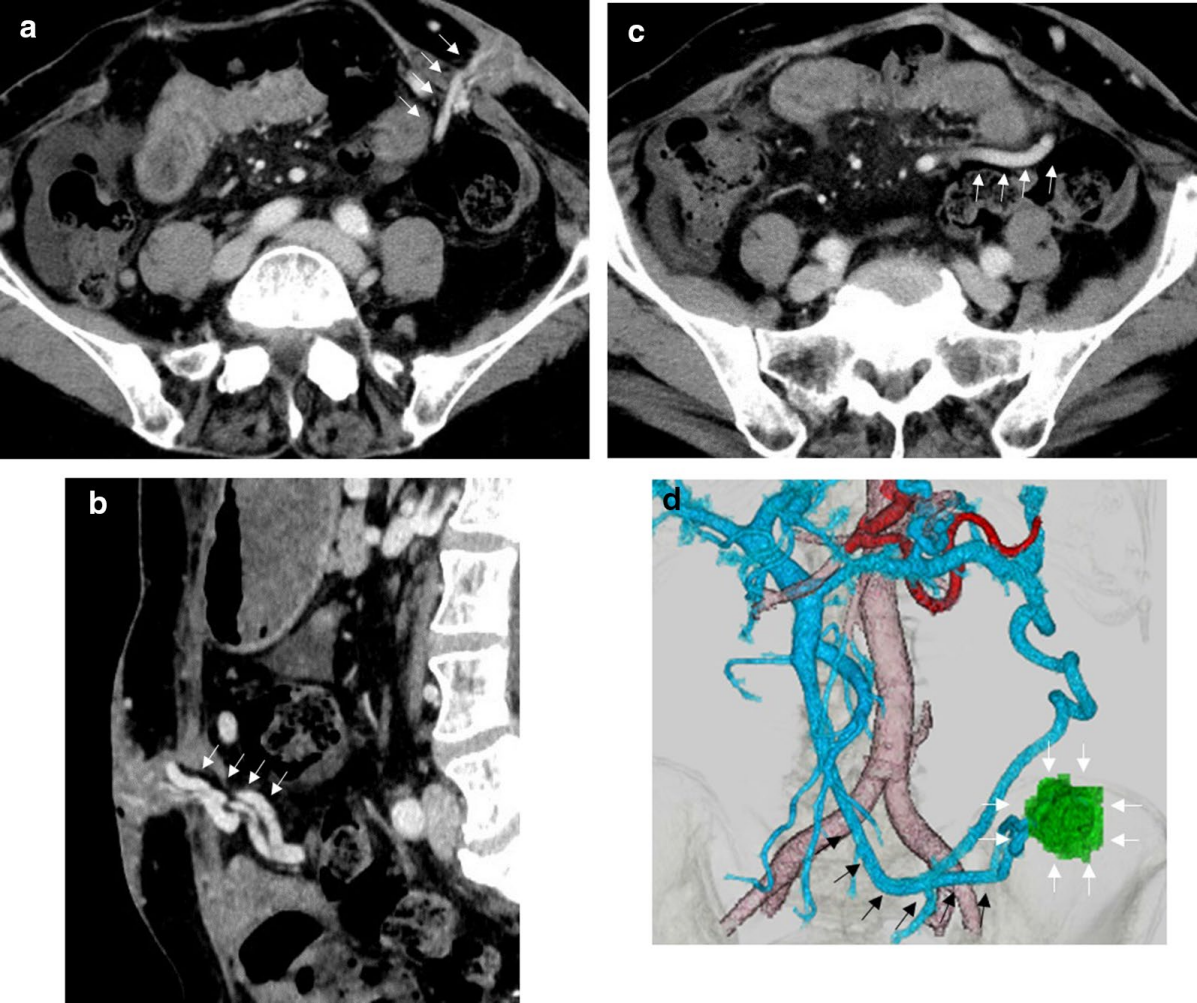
Abdominal CT imaging. a (horizontal slice), b (sagittal slice) Ectopic varices and collateral vessels at the ileal conduit stoma (arrow). c The varices were fed by superior mesenteric vein (arrow). d Three-dimensional reconstruction showed the ileal conduit stomal varices (white arrow) fed by the superior mesenteric vein (black arrow)
